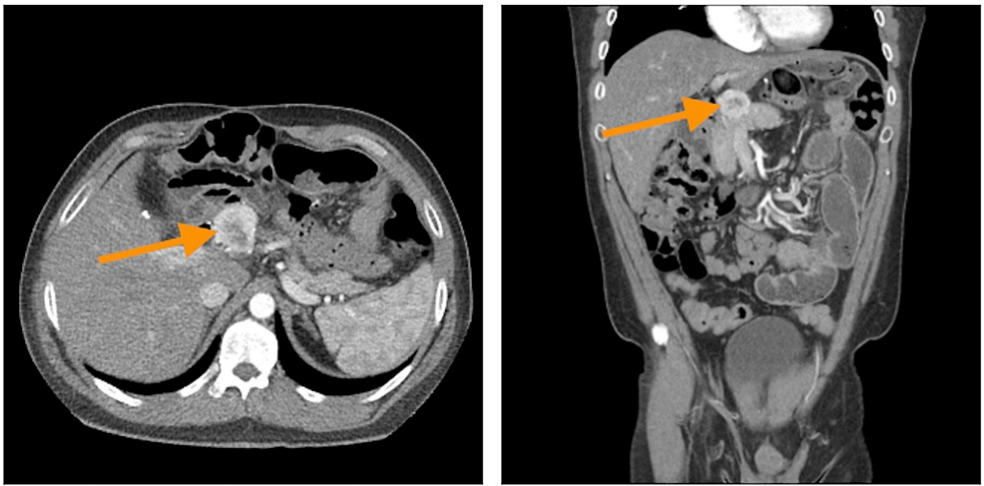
Computed tomography scan of the abdomen with contrast Axial and coronal computed tomography images with contrast are seen above. The orange arrow in each image delineates the tumor location. These images were taken roughly five years after the original symptom onset
